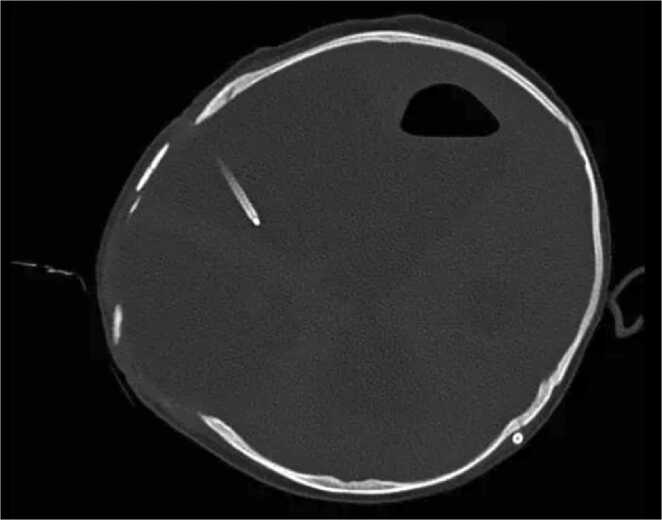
Axial brain CT showing a ventricular shunt, focal skull defect, and a hypodense intracerebral lesion with surrounding edema, consistent with cerebral abscess due to *Neoscytalidium dimidiatum*.
